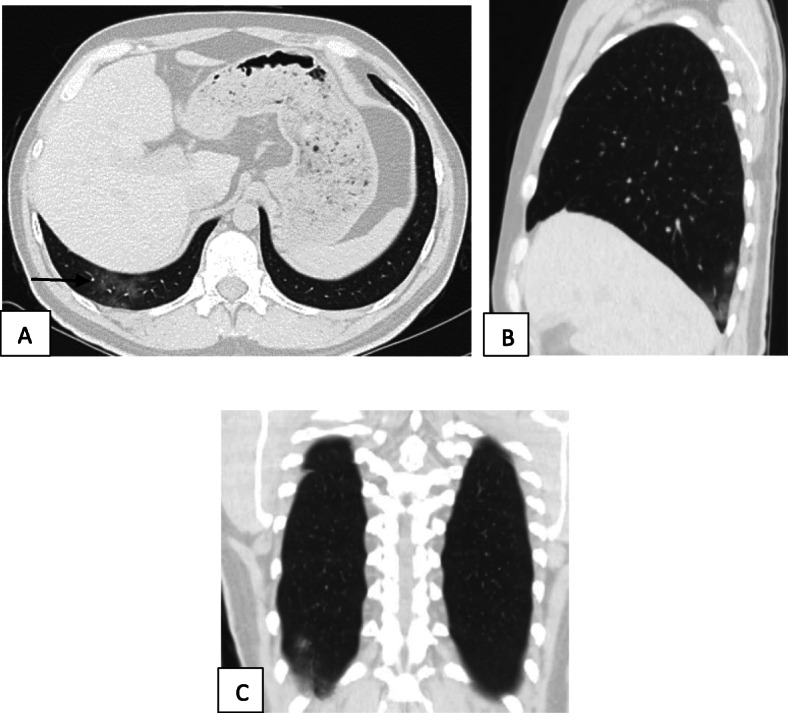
Unenhanced chest CT of a 38-year-old male patient with COVID-19. a An axial lung window image shows the right peripheral subpleural area of ground glass opacity [arrow]. b Same finding on sagittal and c coronal reformatted images. CT score is 1
